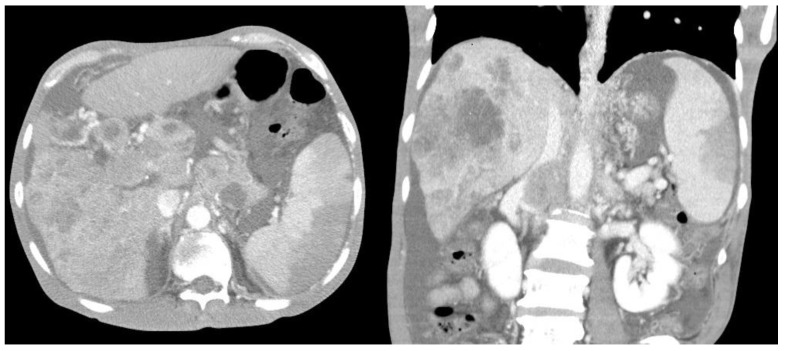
Splenic infarction in a patient with hepatocellular carcinoma. Contrast-enhanced abdominal CT reveals a wedge-shaped splenic hypodensity consistent with infarction, an ill-defined heterogeneous hypodense mass in the right hepatic lobe, portal vein thrombosis, and ascites.
